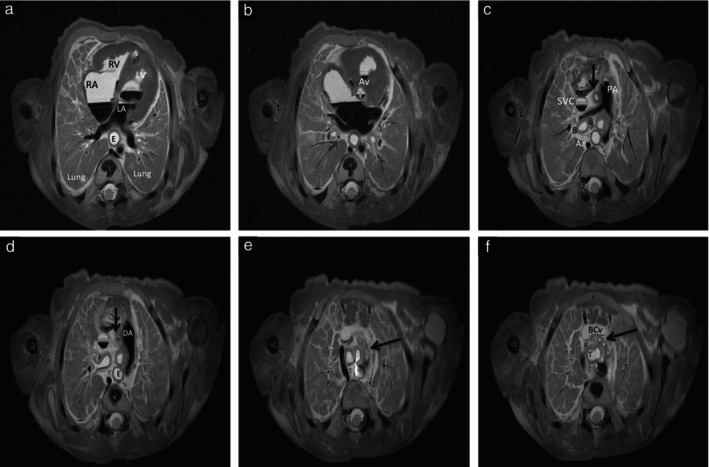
Fetal virtopsy using 7‐Tesla turbo spin‐echo high‐resolution T2‐weighted magnetic resonance imaging in axial plane in fetus at 18 weeks' gestation following termination of pregnancy for cardiac malformation (Case 6). (a) Left ventricle (LV) hypertrophy and reduction in cavity size. (b) Normal appearance of aortic valve (Av). (c) Preductal coarctation of the aorta (arrow). (d) Dilated appearance of ductus arteriosus (DA), probably compensatory. (e) Aortic arch with narrowed aspect (arrow). (f) Three branches (arrow) emerging from aortic arch. Az, azygos vein; B, bronchus; BCv, left brachiocephalic vein; E, esophagus; LA, left atrium; PA, pulmonary artery; RA, right atrium; RV, right ventricle; SVC, superior vena cava; T, trachea.
